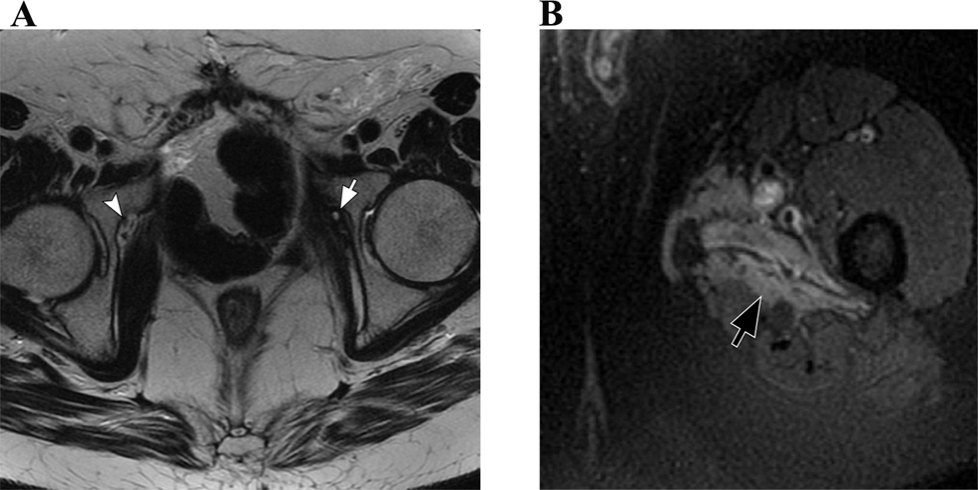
Obturator neuropathy. Patient with a previous left supraacetabular fracture 4 years ago develops left obturator neuropathy. (A) Axial T2-weighted image shows complete obliteration and fibrous tissue surrounding the left obturator nerve (white arrow). The right obturator nerve is normal (arrowhead). (B) Axial T2-weighted image with fat suppression of the proximal thigh. There is muscle edema in the left adductor muscles (black arrow), related to denervation
